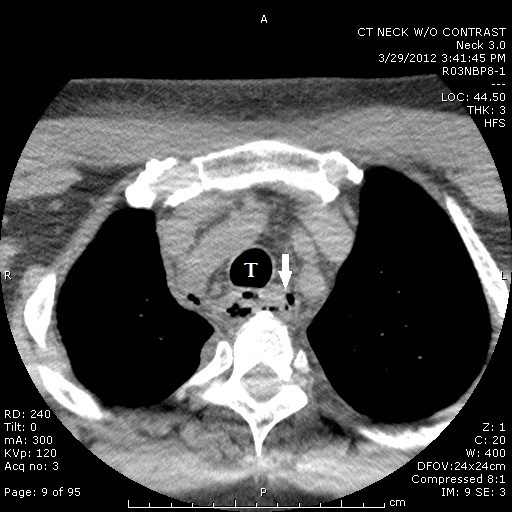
Computed tomography (CT) of the neck showing extensive edema and air within the upper mediastinum (arrow) tracking adjacent to trachea (labeled T).
